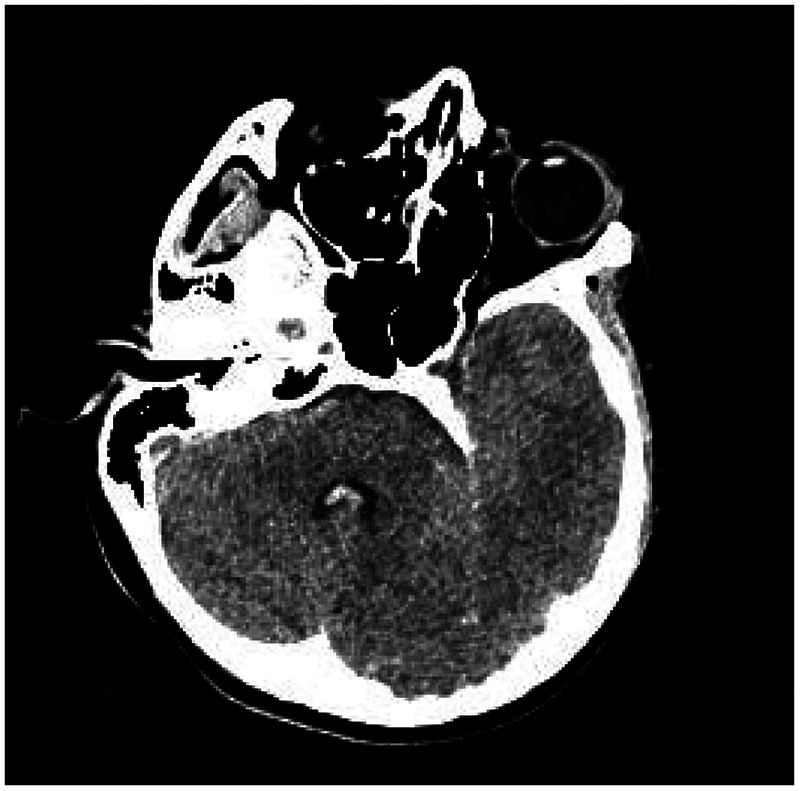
CT shows subarachnoid hemorrhage predominantly within the basal cisterns, with minimal intraventricular hemorrhage.
